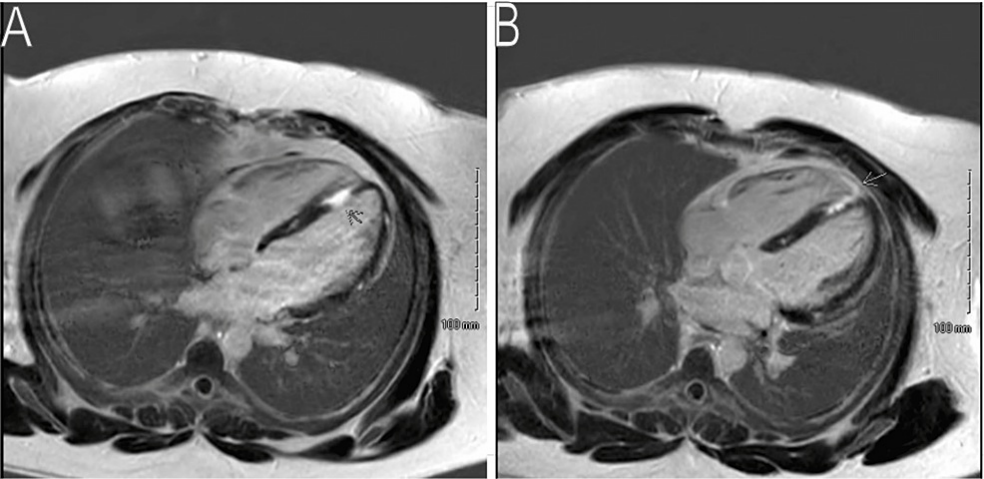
Cardiac magnetic resonance imaging findings (A and B), which demonstrate subepicardial enhancement suggestive of myocarditis (arrows)
